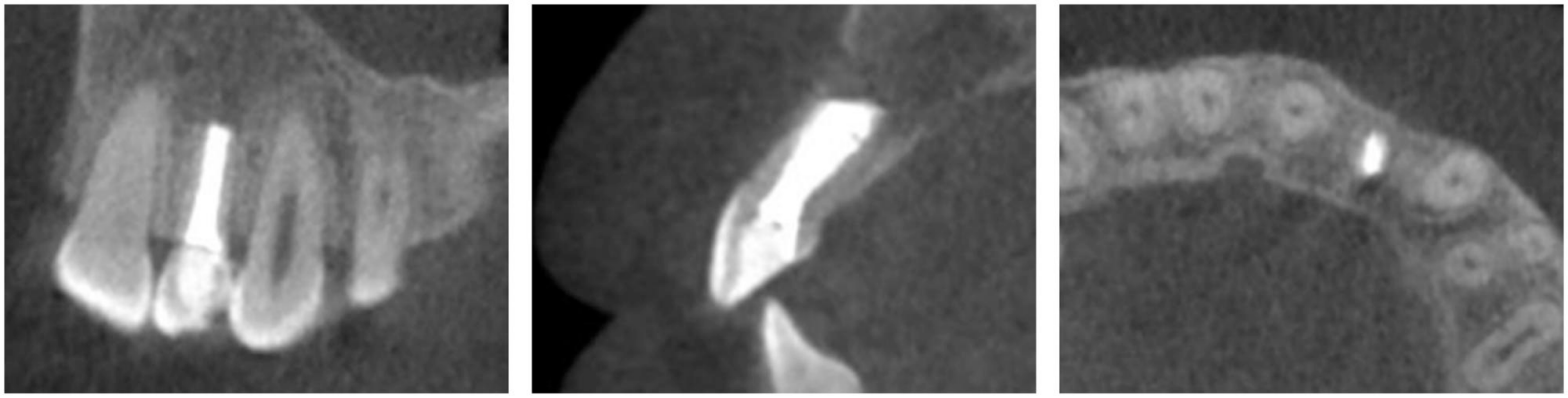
CBCT coronal, sagittal and axial views at postoperative month 6.
